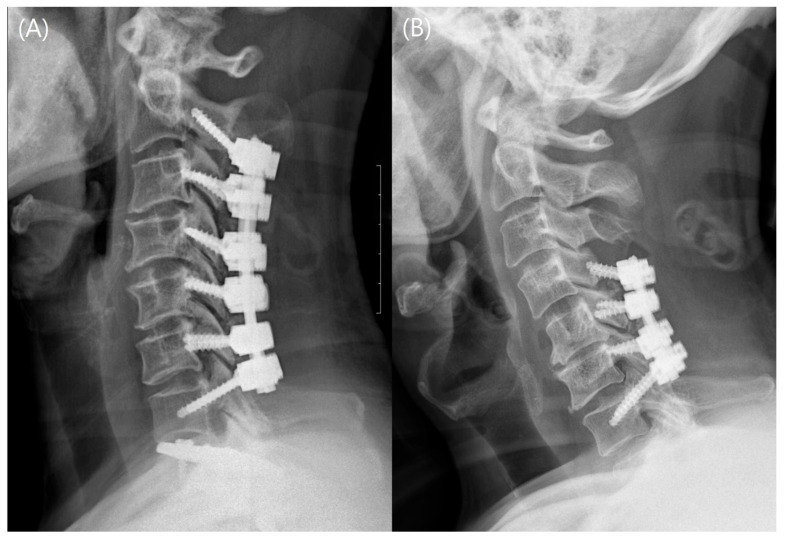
Representative cases. (A) Postoperative 1-year cervical lateral radiograph of an EBF patient. No apparent screw failure was observed on follow-up radiograph. (B) Postoperative 1-year cervical lateral radiograph of an RF patient. The proximal screw failure is confirmed on follow-up radiograph.
